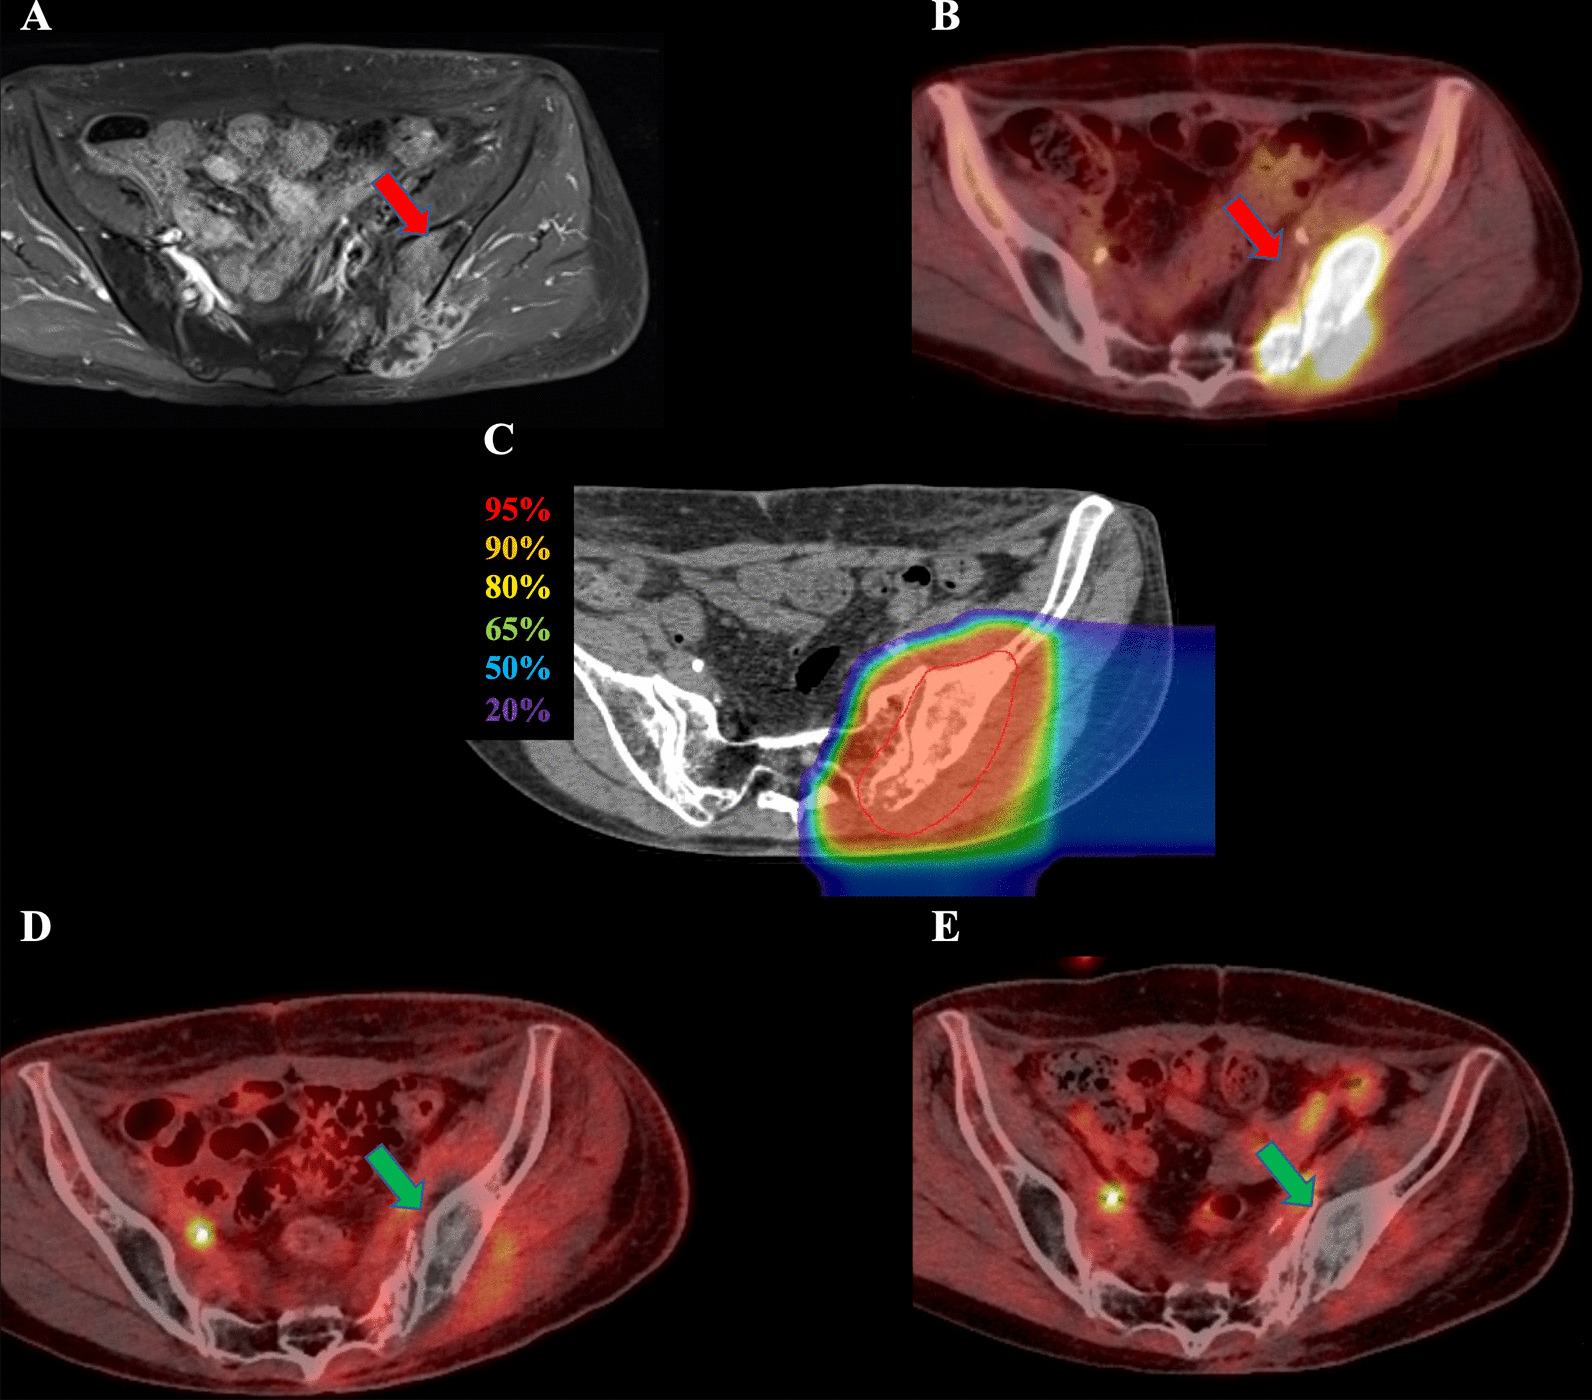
Radiological images before and after carbon-ion radiotherapy (C-ion RT) and dose distribution of Case 1. a Contrast-enhanced magnetic resonance imaging (MRI) before C-ion RT. The tumor (50 × 80 × 95 mm) was located in the left iliac bone and had good contrast enhancement (red arrow). b 2-deoxy-2-[^18^F]fluoro-D-glucose-positron emission tomography (FDG-PET) before C-ion RT. The red arrow shows the tumor with abnormal FDG uptake. c Dose distribution on axial computed tomography images. The area within the red outline is the gross tumor volume of the osteosarcoma. The 95% (red), 90% (orange), 80% (yellow), 65% (green), 50% (blue), and 20% (purple) isodose curves are highlighted (100% = 70.4 Gy [relative biological effectiveness]). d FDG-PET 1 year after C-ion RT. FDG uptake was decreased compared to that before treatment (green arrow). (E) FDG-PET 5 years after C-ion RT. FDG uptake was decreased compared to that before treatment (green arrow)
